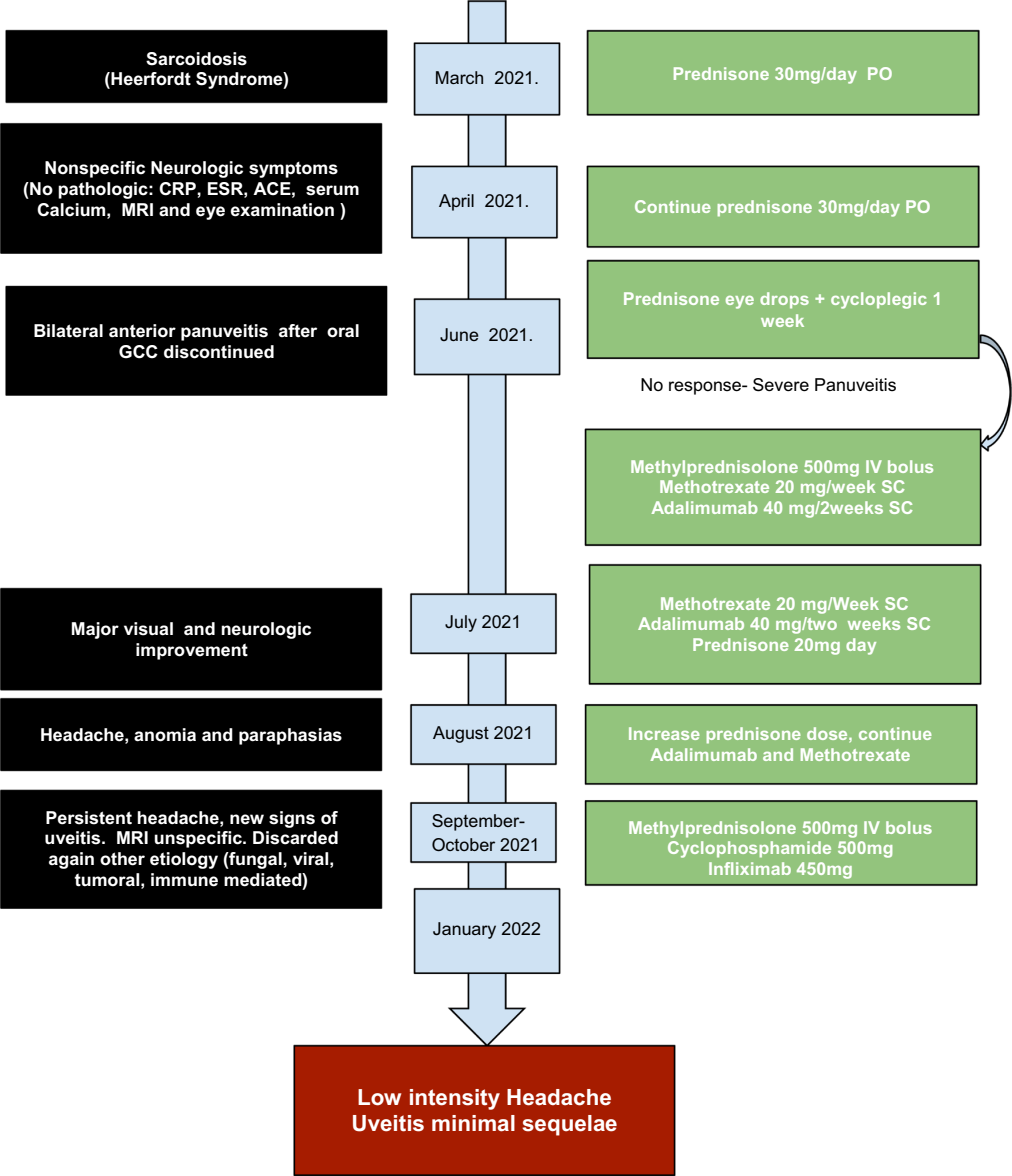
Timeline of the disease process. *GCO* oral glucocorticoids, *MRI* magnetic resonance imaging, *CRP* C reactive protein, *ESR* erythrocyte sedimentation rate, *ACE* angiotensin converting enzyme
